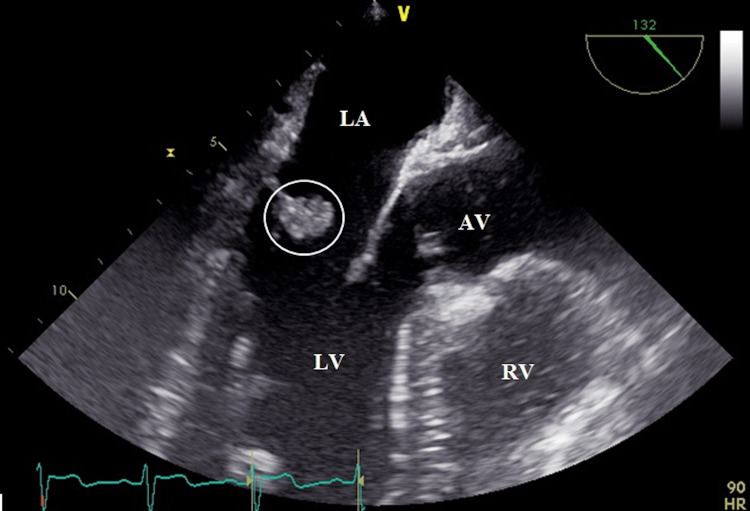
Transoesophageal echocardiography demonstrating an echodensity on the atrial aspect of the posterior mitral valve leaflet AV: aortic valve; LA: left atrium; LV: left ventricle; RV: right ventricle.
